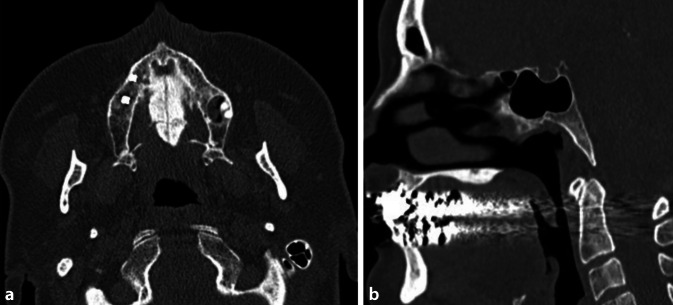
Example of an excluded patient. Exclusion was based on palate thickening due to torus palatinus. a Axial CT of bilobulated high-density masses from the midline of the hard palate (torus palatinus). b Shows the typical exostosis of torus palatinus in sagital view
